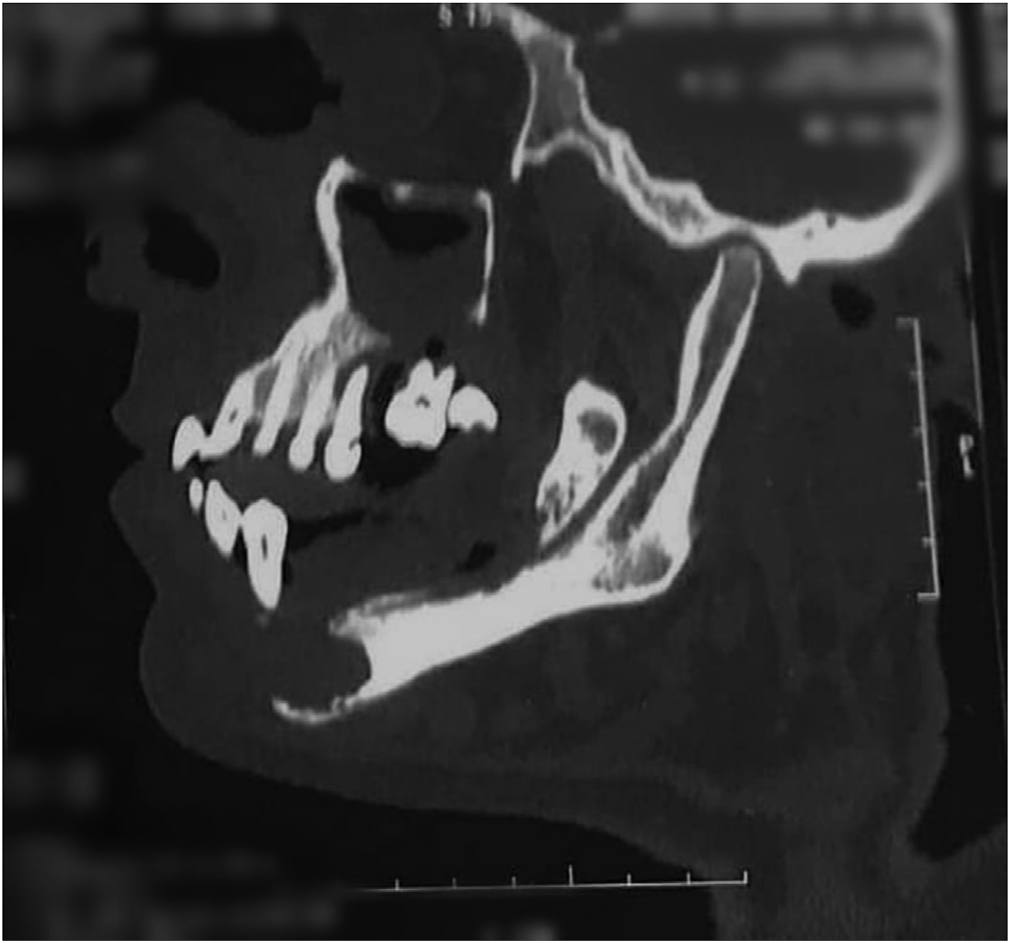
CT scan with a sagittal section showing bifocal (maxillary and mandibular) osteolytic lesions.
